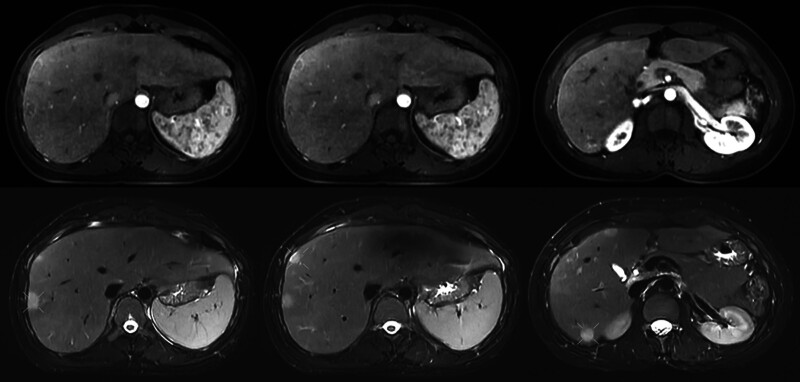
Upper abdominal magnetic resonance imaging with disodium gadosenate in November 18, 2023: T1-weighted image arterial enhancement phase and T2-weighted image nonarterial enhancement phase.
